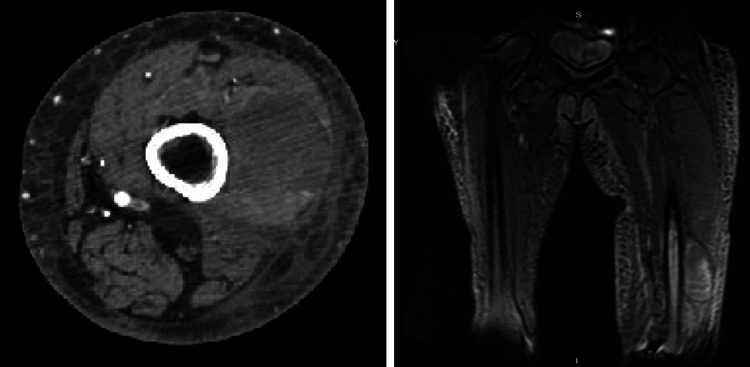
Computed tomography angiography (CTA) of the left lower extremity (left) and magnetic resonance imaging (MRI) of the femur (right) demonstrating a left vastus lateralis hematoma.
